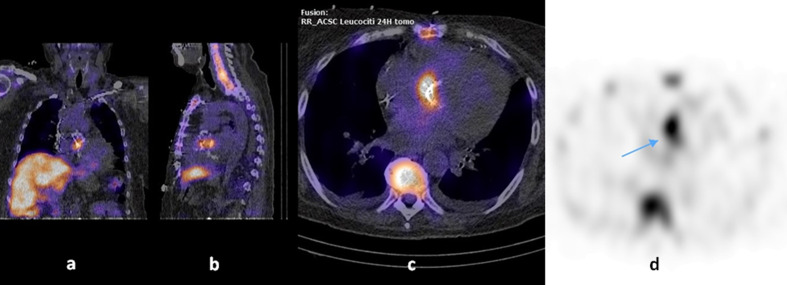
Tc-99m HMPAO WBC SPECT images of a *
